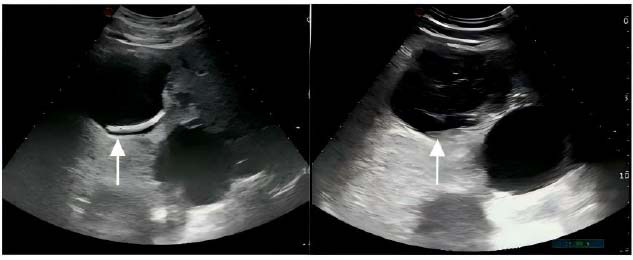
Failed case: Ultrasound image of a 53-year-old man with a hepatic cyst. The initial volume of the hepatic cyst in the left image was
